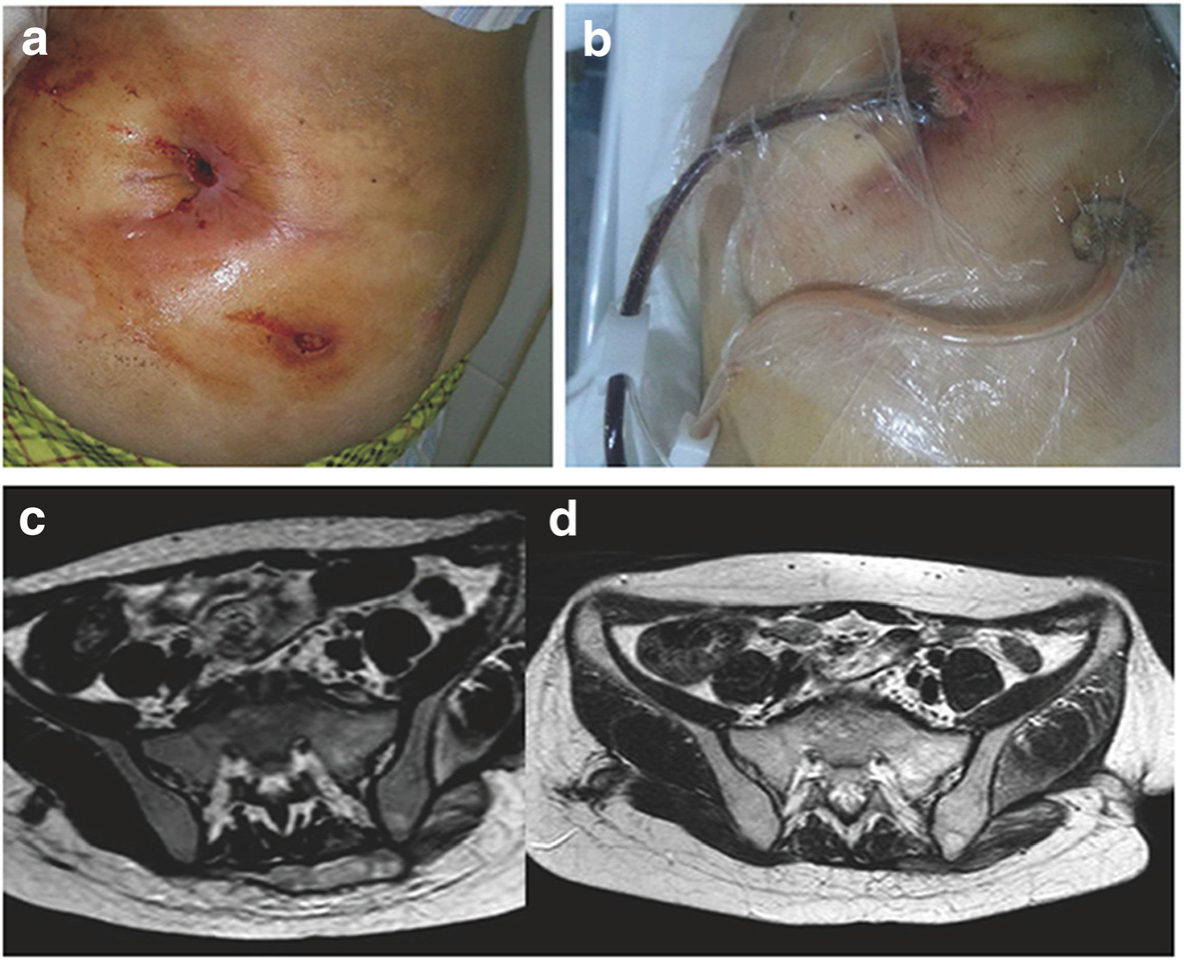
a Sinus tracts in the left buttock. b Management with NPWT. c MRI of the sacroiliac joints: erosive changes and abscess involving the right sacroiliac joint and sinuses in the left buttock. d MRI demonstrating healed abscesses and solid bony fusion 36 months after NPWT and anti-tubercular therapy
